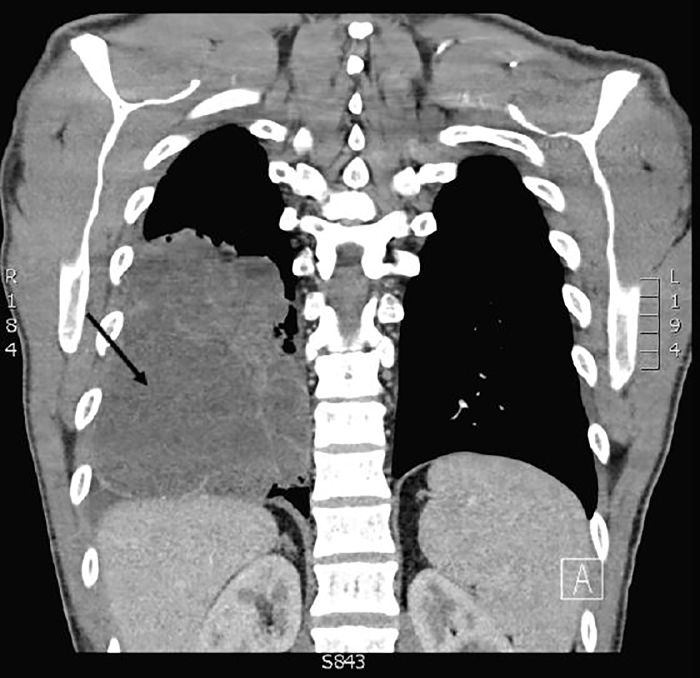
Chest computed tomography demonstrating a right-sided pulmonary abscess (arrow).
